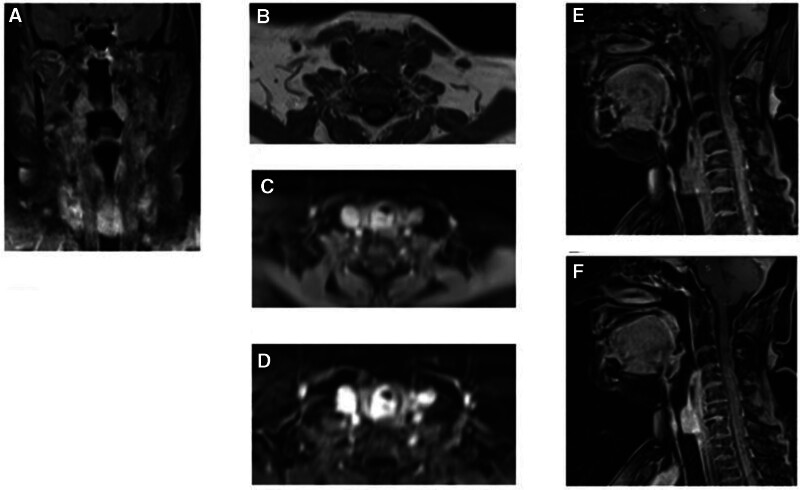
MRI of neck and thorax; coronal T2 (A), axial T1 (B), axial T1 fat sat with contrast (C), subtraction (D), sagittal T1 fat sat (E), and sagittal T1 fat sat with contrast (F) demonstrating a hypervascular mass on the posterior tracheal wall with luminal narrowing. The mass shows iso-intensity on T1W, hyper-intensity on T2W, and marked enhancement on contrast-enhanced images. MRI = magnetic resonance imaging.
